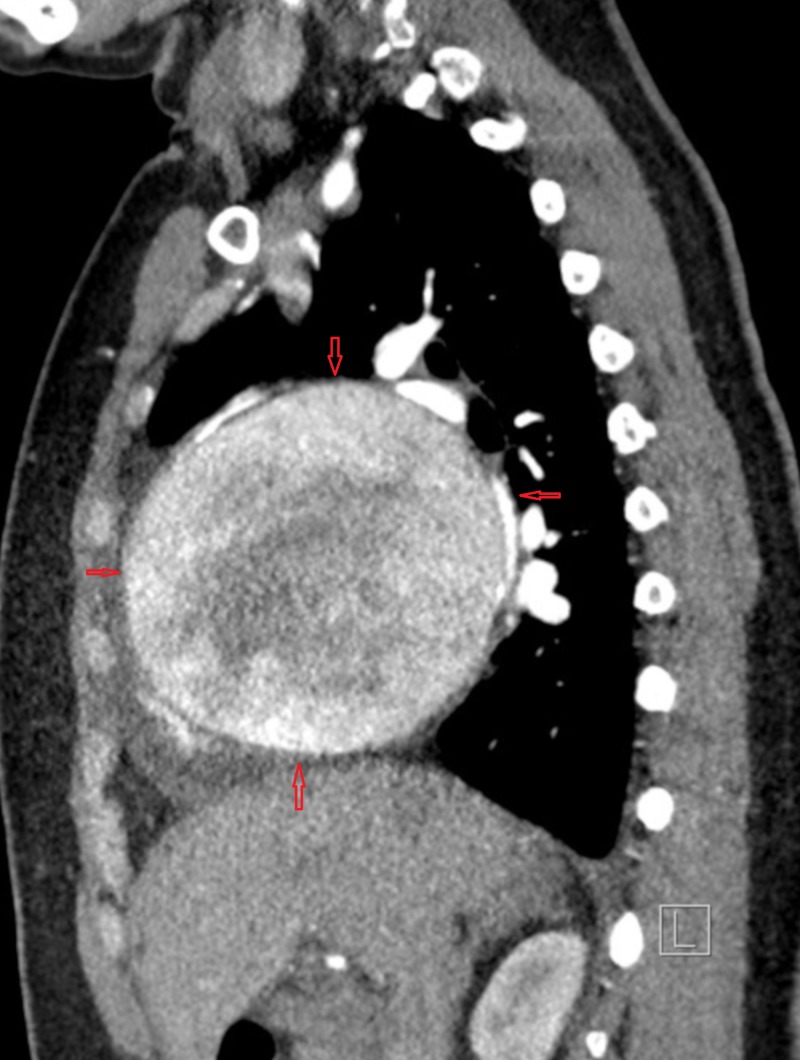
CT coronary showing a giant coronary artery aneurysm CT: computed tomography
